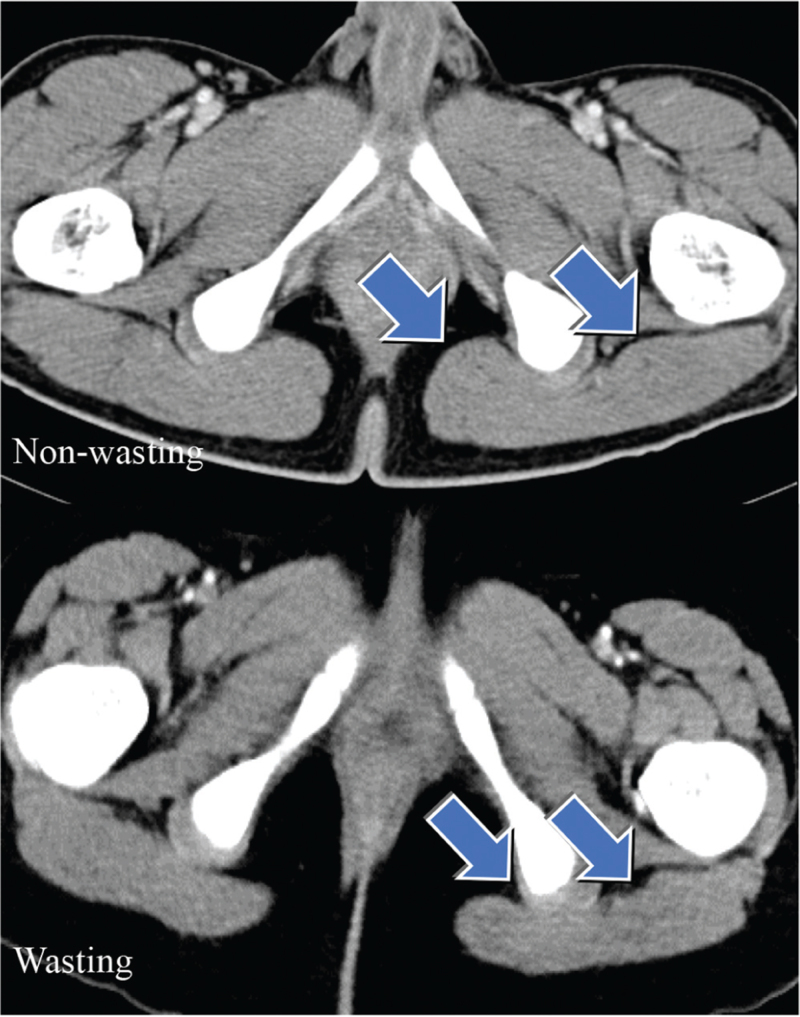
Left gluteus maximus muscle (arrow) surface areas of the patients on a single image of computed tomography at the most thickened level of the left ischiofemoral space.
